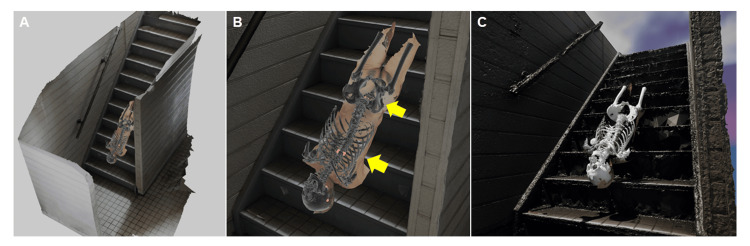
Scene reconstruction by integration of 3D models of postmortem CT data, surface information, and staircases. (A) Overall view. (B) Close-up view. As indicated by the yellow arrows, the width of the staircase corresponds approximately to the width of the body fractures. (C) Virtual reality-based scene reconstruction. For clarity, the bones are shown in white.
